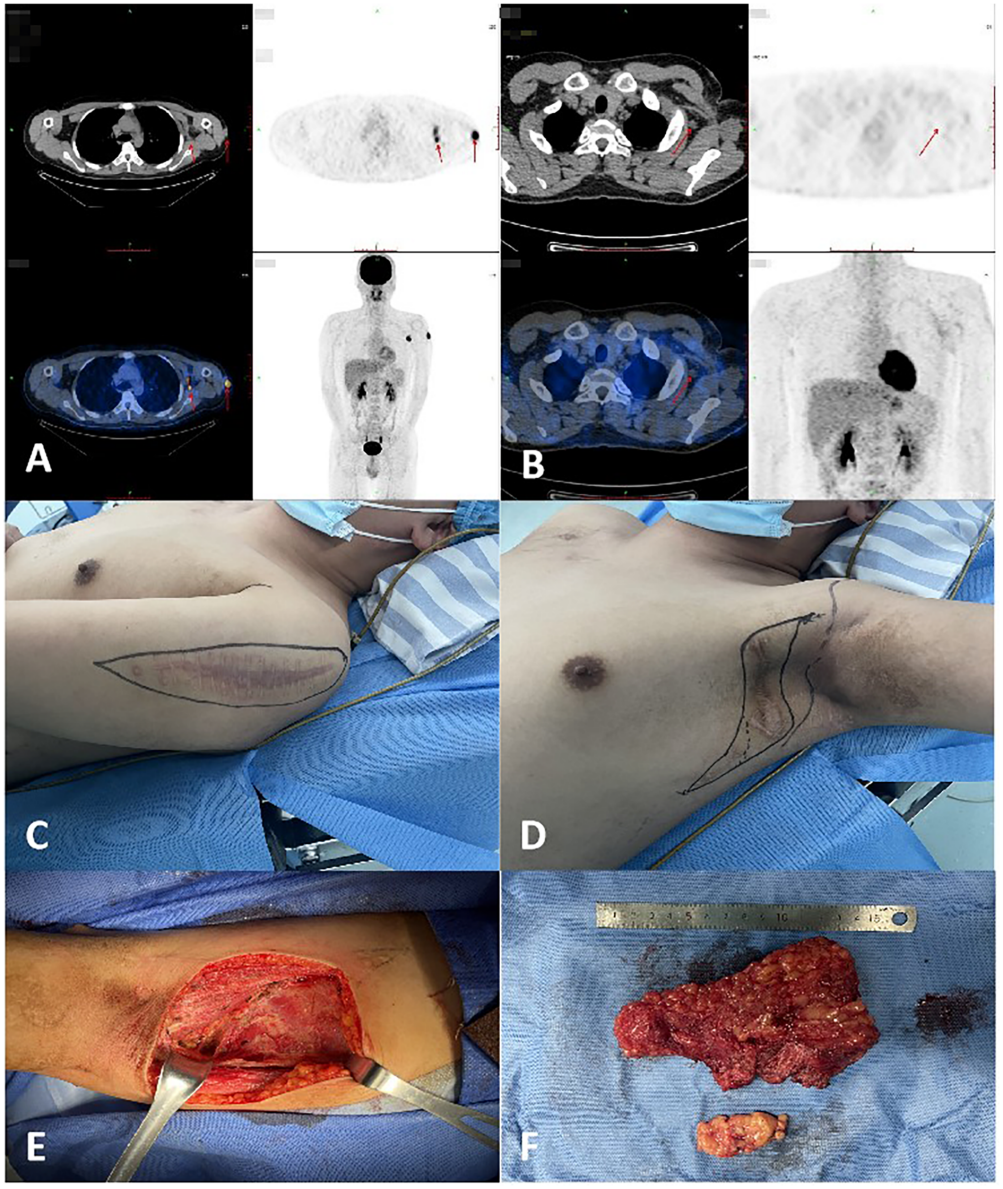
(A) The patient’s PET-CT results indicated lymph node metastasis in the left axilla in January 2023. (B) The patient’s PET-CT results revealed left axillary lymph node metastases in April 2023. (C) Preoperative photograph of the recurrent tumor mass in the patient’s left upper arm awaiting excision. (D) Preoperative photograph of the metastatic lymph nodes in the patient’s left axilla awaiting dissection. (E) Intraoperative photos of the patient. (F) Photos of the removed mass.
